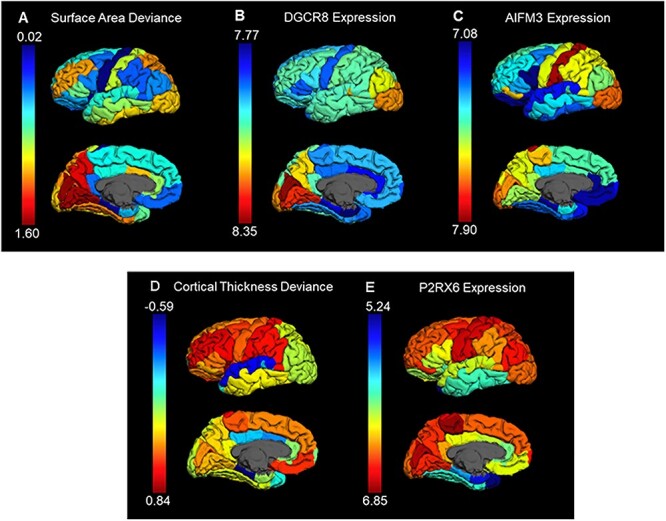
Variation across 34 left hemisphere cortical regions in: A) Z-score surface area deviance (ΔSA) severity in 22q11DS patients relative to controls (higher Z-score indicates region with greater reduction in SA in 22q11DS); B) *DGCR8* expression; C) *AIFM3* expression; D) Z-score cortical thickness deviance (ΔCT) severity in 22q11DS relative to controls (higher Z-score indicates region with greater increase in CT in 22q11DS); and E) *P2RX6* expression. Expression of *DGCR8* and *AIFM3* were significantly associated with ΔSA in 22q11DS and expression of *P2RX6* was significantly associated with ΔCT in 22q11DS.
